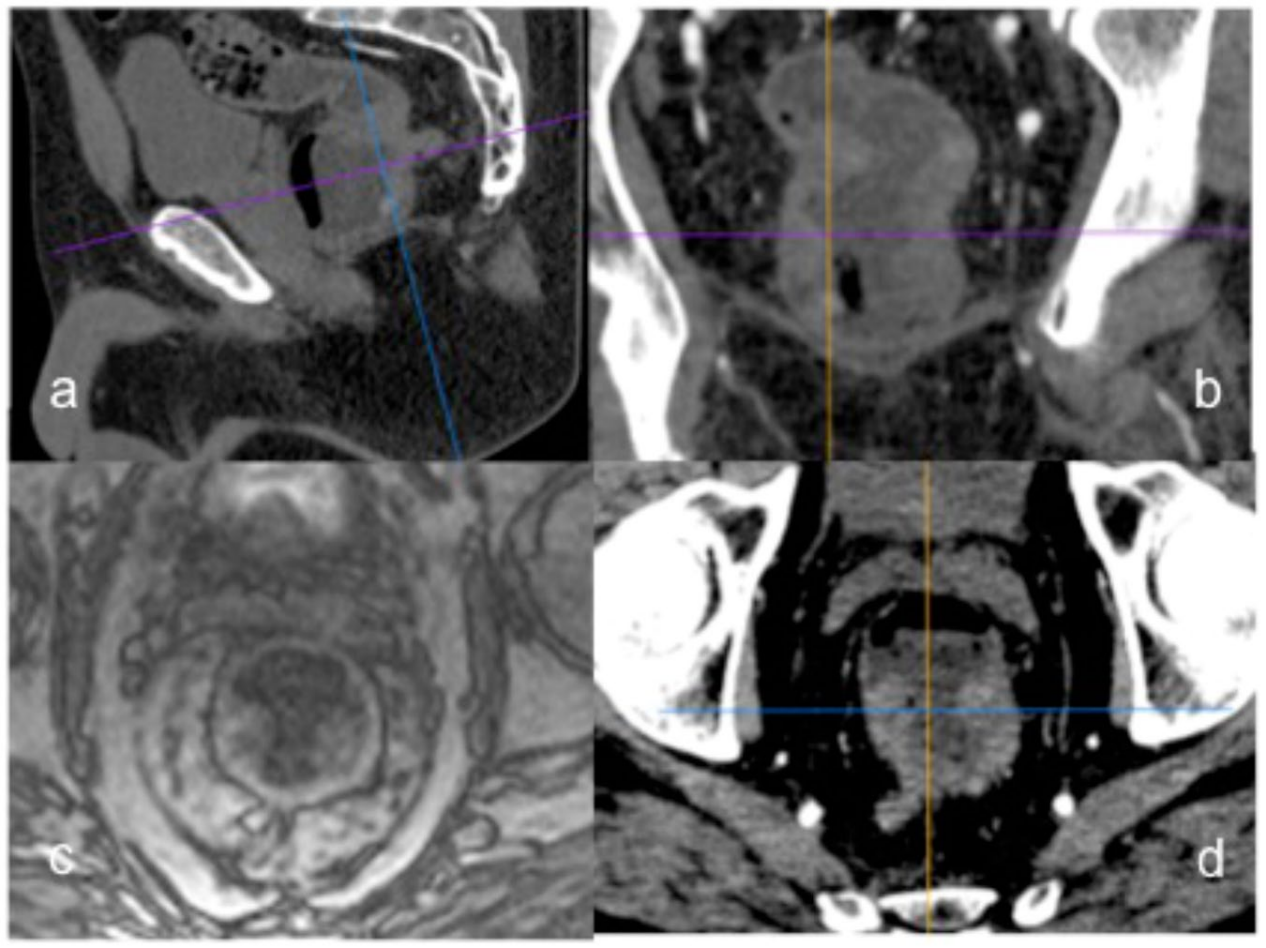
Example of corresponding rectal cancer tumour areas in MR and CT and localization of the investigated tumour area within the pelvis: (a) Sagittal view of the rectal carcinoma and localization of the the representative tumour area investigated with perfusion analysis. (b) Coronal view of the rectal carcinoma and localization of the representative tumour area investigated with perfusion analysis. (c) Paraaxial MR image from the T1 weighted TWIST angiographic stack perpendicular to the rectum lumen, with representative semicircular tumour area at 3 to 9 o’clock suitable for tumour perfusion analysis. (d) Paraaxial CT image from the dVPCT stack perpendicular to the rectum lumen, with the corresponding representative semicircular tumour area at 3 to 9 o’clock suitable for tumour perfusion analysis.
